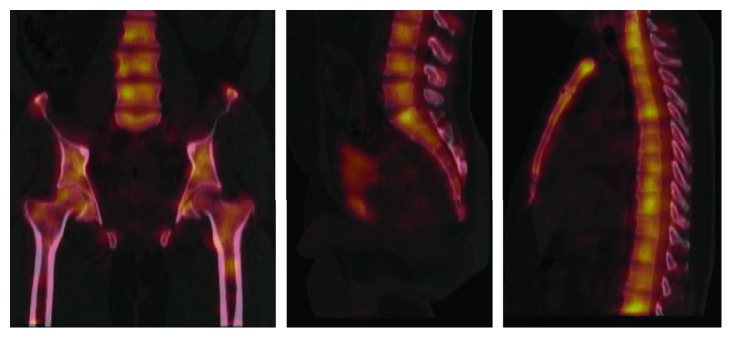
SPECT-CT showing diffuse bone and bone marrow lesions.
